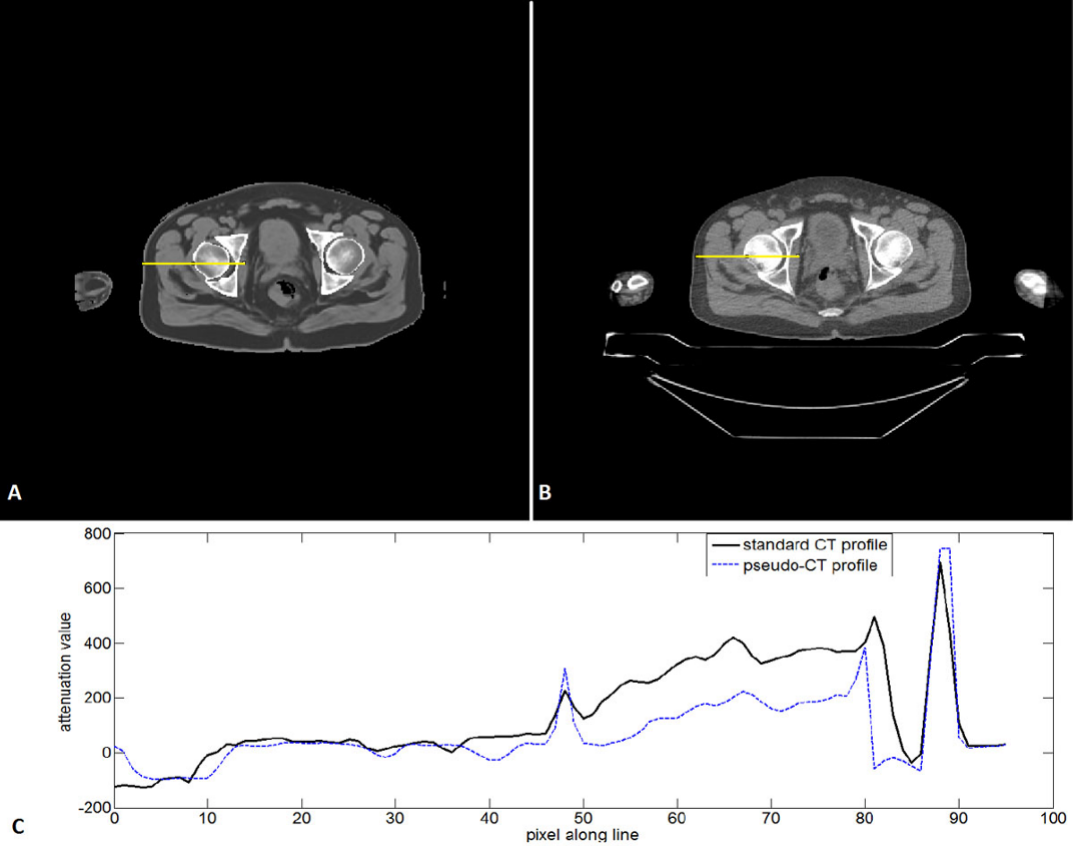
An example of a generated pseudo-CT slice (A) and its corresponding standard CT slice (B). Profiles over the line are plotted underneath (C).
